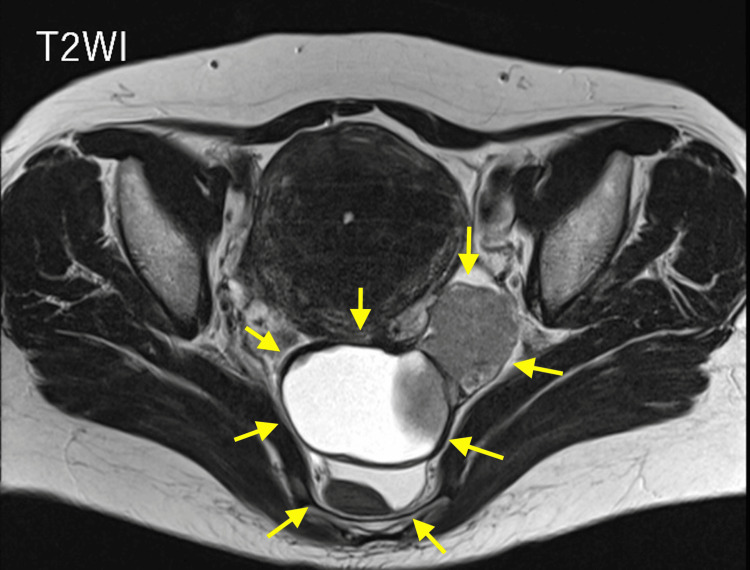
Pelvic contrast-enhanced MRI. The cystic component was considered to be a lesion containing a blood component such as an endometriotic cyst. The solid component was almost homogeneously visualized with a slightly higher signal than the muscle, and the contrast effect was good and homogeneous.
